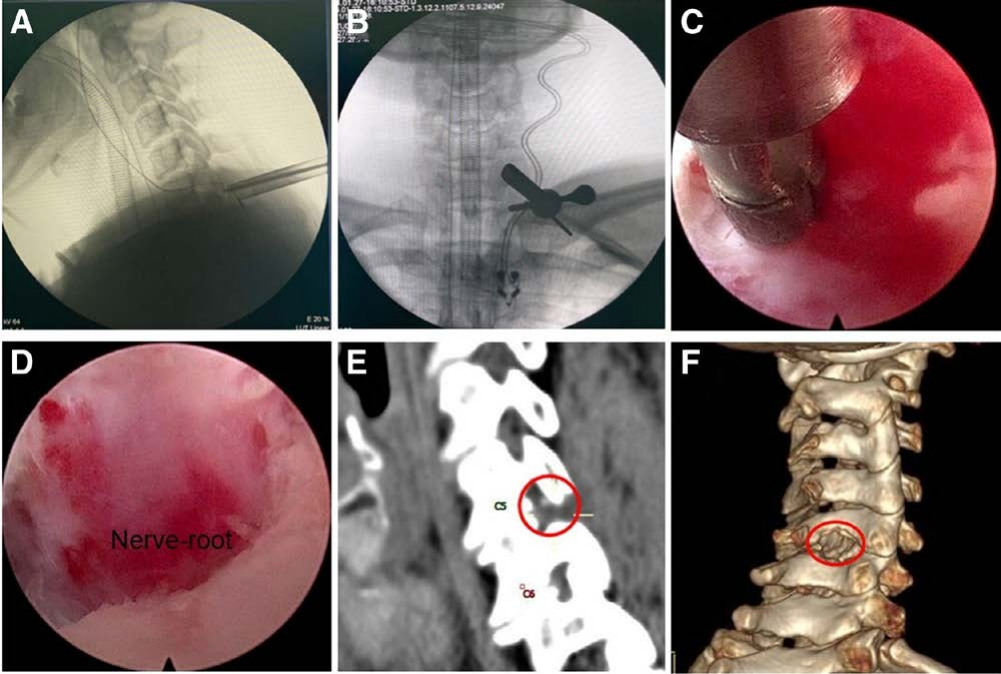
The K-wire was inserted through the safe operating area and anchored into the objective articular process, and serial dilators and endoscopic were established (A, B). Endoscopic radiofrequency electrocoagulation for hemostasis (C). The nerve root was completely decompressed (D). The sagittal view of CT (E) and three-dimensional reconstruction (F) demonstrate the enlargement of the intervertebral foramen. CT = computerized tomography.
